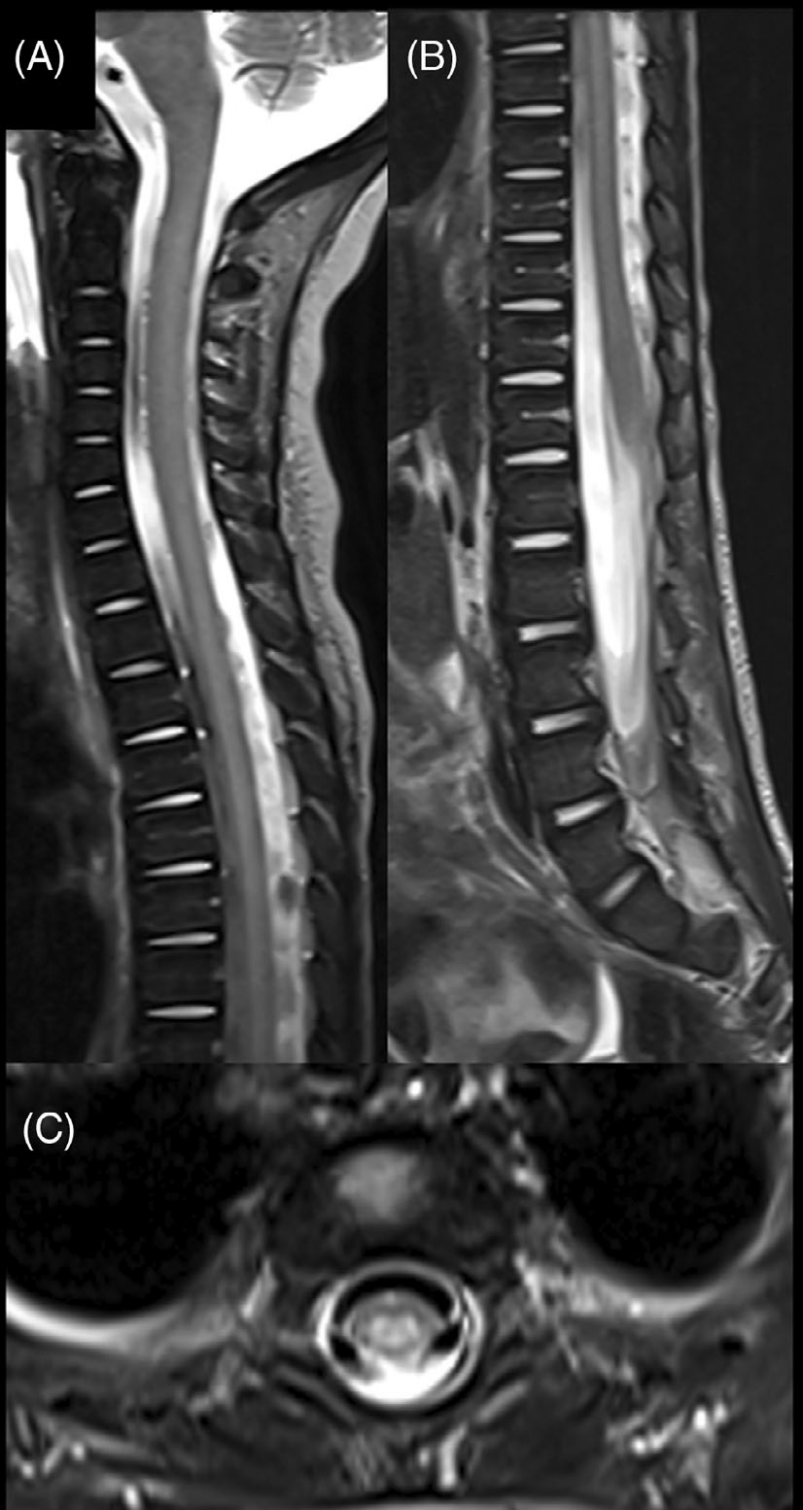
MRI of the spine after treatment. (A, B) Sagittal T2‐weighted images demonstrate abnormal T2 hyperintensity involving nearly the entire spinal cord. (C) Axial T2‐weighted image shows the central distribution of the signal abnormality in the spinal cord with relative sparing of the periphery of the cord.
